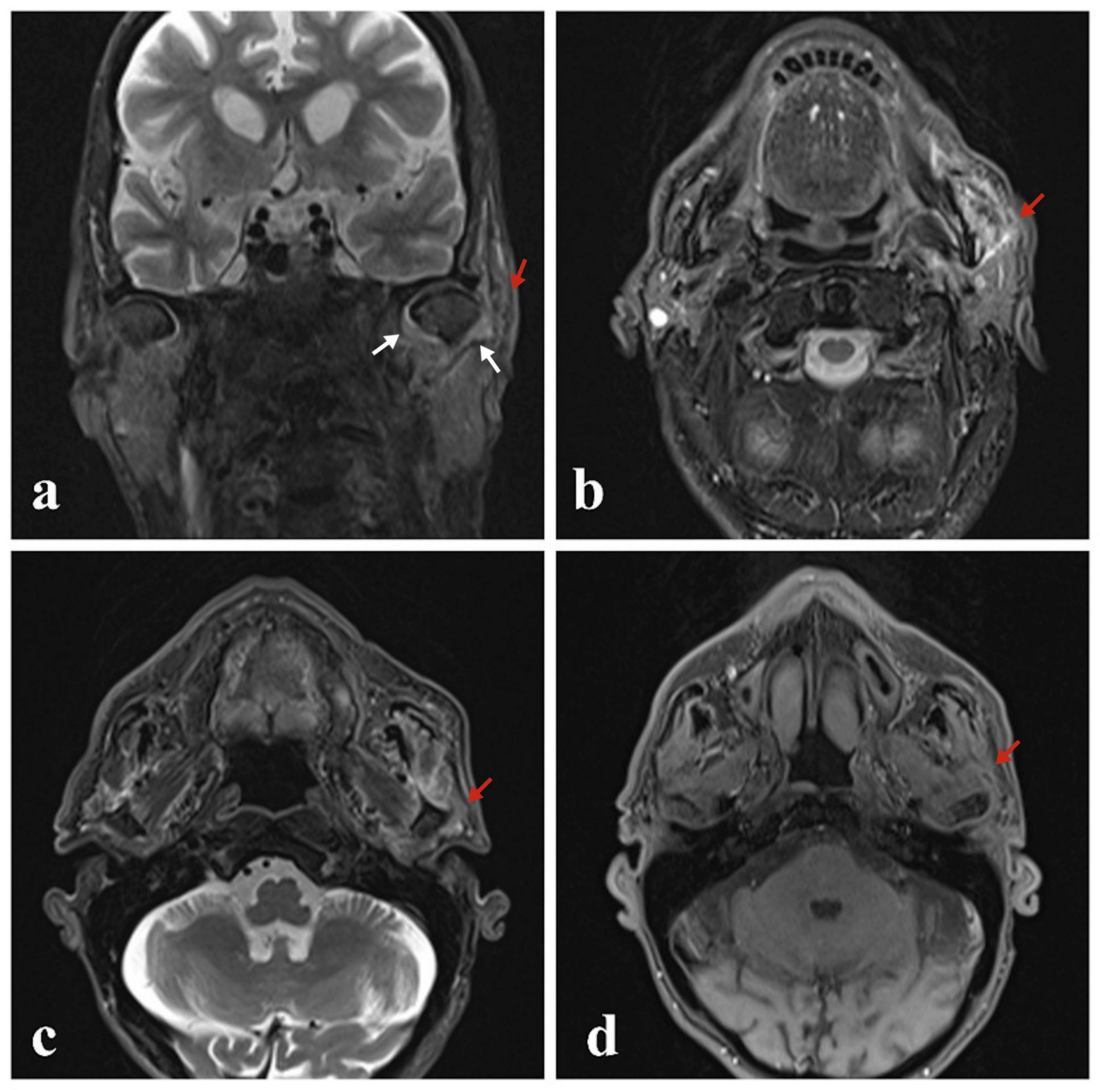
Images of facial magnetic resonance imaging Edema and increased thickness were observed in the masticatory muscles, especially the masseter muscle, in the coronal T2 FS (a), axial T2 FS (b,c), and axial T1 FS (d) images (red arrows). There was a minimal fluid increase in the left temporomandibular joint in the coronal T2 FS (a) images (white arrows).
